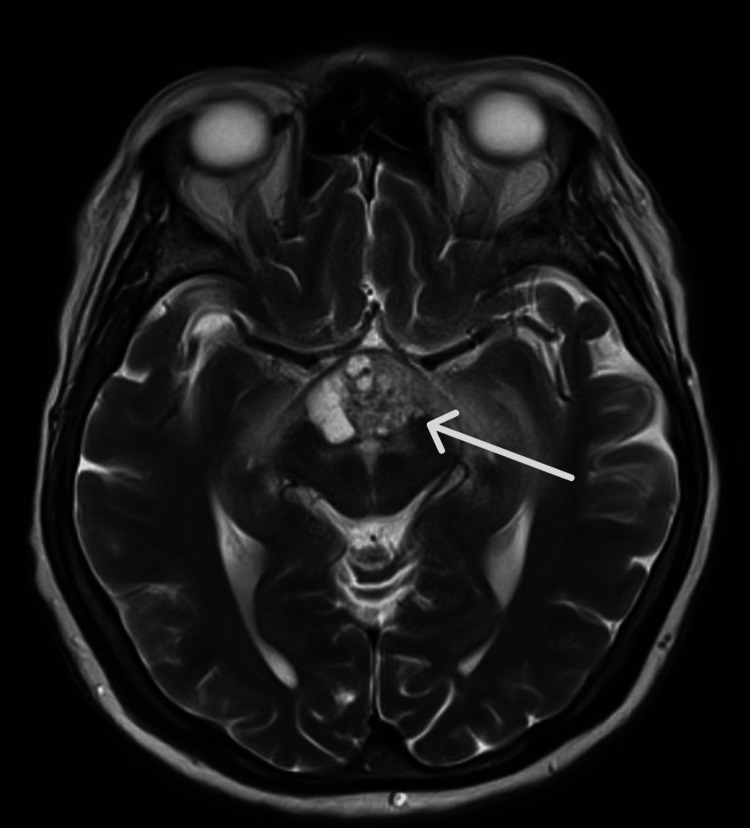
Non-contrast MRI brain with (axial T2) MRA performed on April 26, 2024 Relevant findings include a midline suprasellar hypothalamic heterogeneous solid-cystic mass, with local mass effect and perilesional edema, as well as apparent extension to the optic chiasm, but no overt sellar involvement. The mass measures up to 30x20 mm in the axial plane and 17 mm in the craniocaudal direction. Susceptibility suggesting hemorrhage is seen in the solid component. The third ventricle floor is indented superiorly. There is edema along the optic radiations, as well as mass effect and edema involving the midbrain and thalami bilaterally. MRA: magnetic resonance angiography
